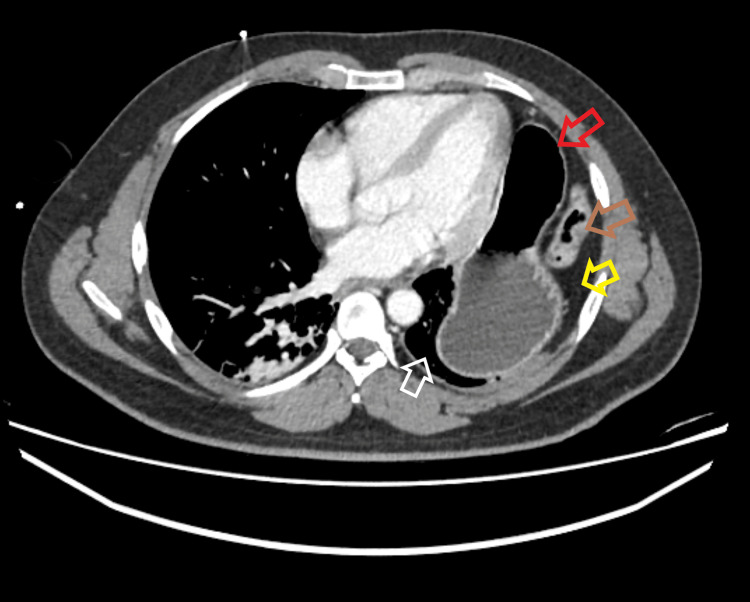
Computed tomography scan done at the initial presentation (axial view) showing the herniated stomach (red arrow), omentum (yellow arrow), and colon (brown arrow), all within the left hemithorax, causing the left lung (white arrow) to be collapsed
